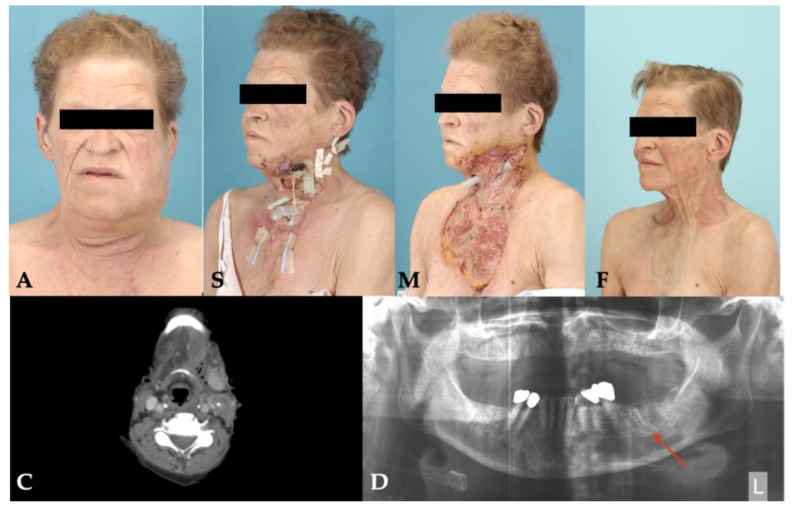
Clinical course and radiographs of patient No. 1: A: admission; S: surgery; M: maximal extent; F: final result; C: CT scan; D: dental focus (empty alveolus).
